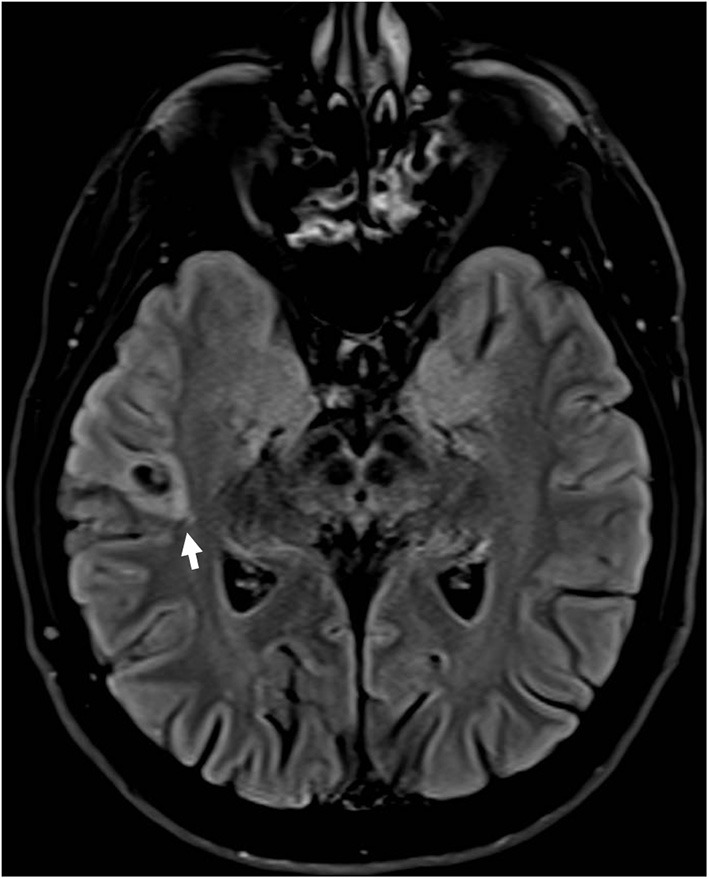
Brain MRI (FLAIR; axial view) in a patient with drug-resistant epilepsy with auditory features showed a small hyperintense lesion in the right lateral temporal cortex surrounded by an area of signal suppression and hyperintensity of the adjacent brain tissue, consistent with a glioneuronal tumor.
